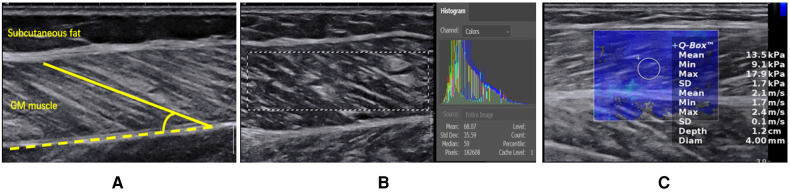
(A) Measurement of muscle fascicle length and PA by gray-scale ultrasound on the long axis of GM. The yellow dotted line indicates the deep aponeurosis of GM, and the yellow solid line indicates the muscle fascicle length. The angle between the muscle fascicle and the deep aponeurosis is PA. (B) EI analysis of GM muscle by using adobe Photoshop cc 2021 software. The mean gray value was shown in the histogram interface. (C) Measurement of the Young's modulus of GM.
